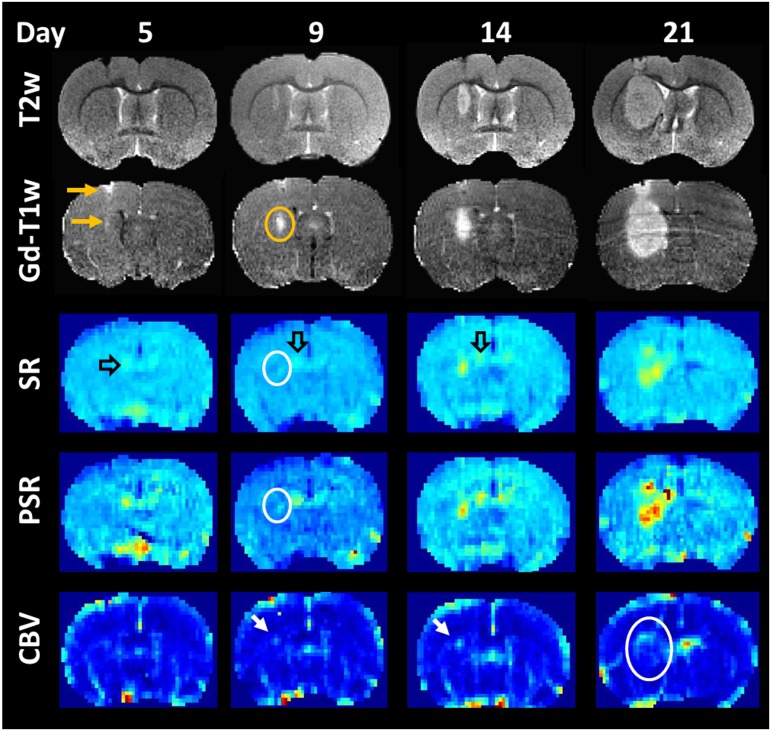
Time course of tumor development after intracerebral injection of 10,000 C6-glioma cells. T2-weighted images (T2w) revealed an increase in tumor mass over time which was accompanied by an increasing area of contrast enhancement (G1-T1w). At day 9 maps of percentage of signal recovery (PSR) and signal recovery (SR) indicated a higher capillary permeability in the tumor center (white ring) extending over time. Higher PSR and SR values were also seen in the region of the choroid plexus (open arrow). Higher cerebral blood volume (CBV) was mainly found at the tumor rim (white arrow and ring).
